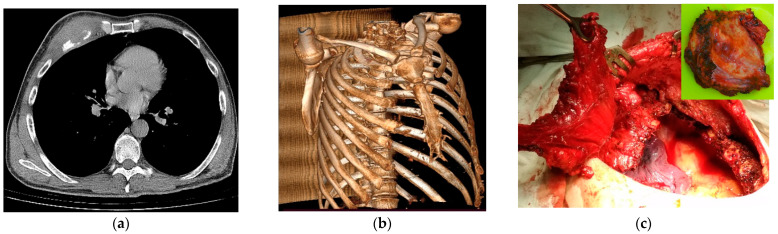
Patient XX, 48 y.o., with anterior chest wall metastatic mass invading ribs on the right from renal cell carcinoma: presurgical (a) axial CT thoracic scan and (b) 3D reconstruction of the chest osteochondral frame, (c) intraoperative view of the *pectoralis major*/*minor* to be excised together with anterior segments of three ribs (3rd–5th).
